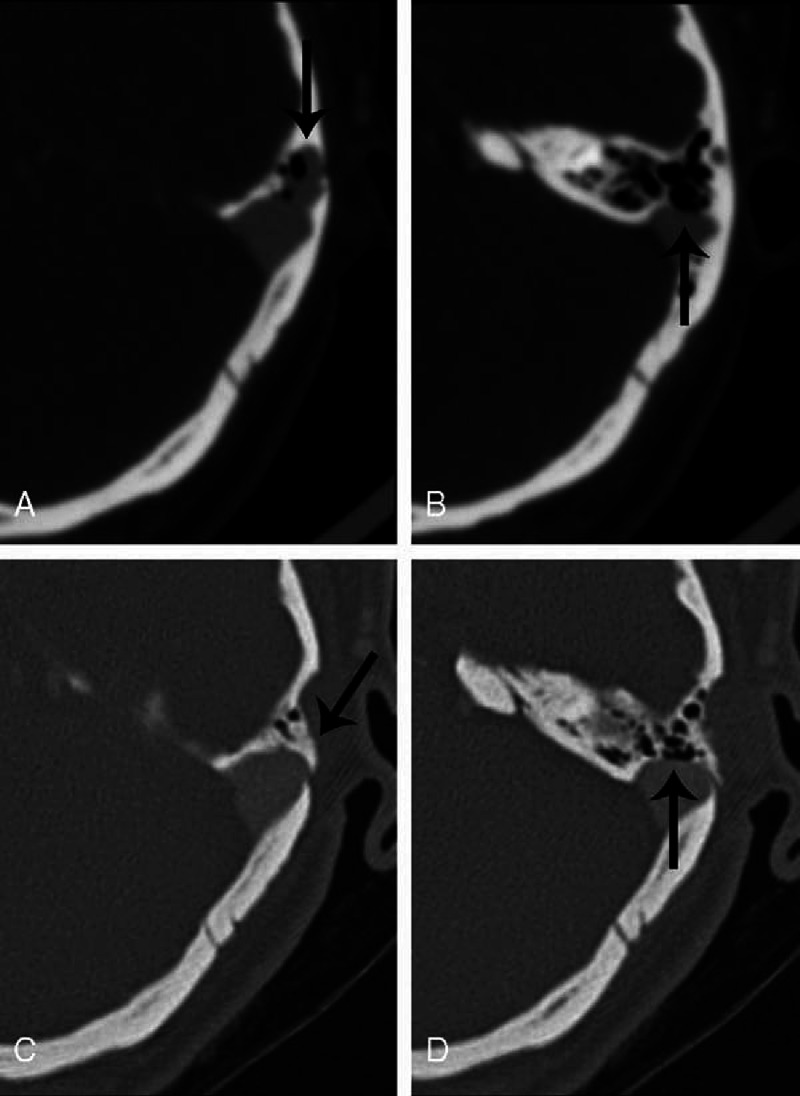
Preoperative (A and B) and postoperative (C and D) CTA images for a patient (Patient 10) experiencing the partial resolution of PT following surgery. A, The diverticulum erodes into the mastoid air cells (arrow). B, In the layer inferior to that of (A), the sigmoid sinus wall is dehiscent (arrow). C, The postoperative CTA image corresponding to the layer of (A) shows that the diverticulum is well eliminated (arrow). D, The postoperative CTA image corresponding to the layer of (B) shows that dehiscence of the wall remains (arrow).
